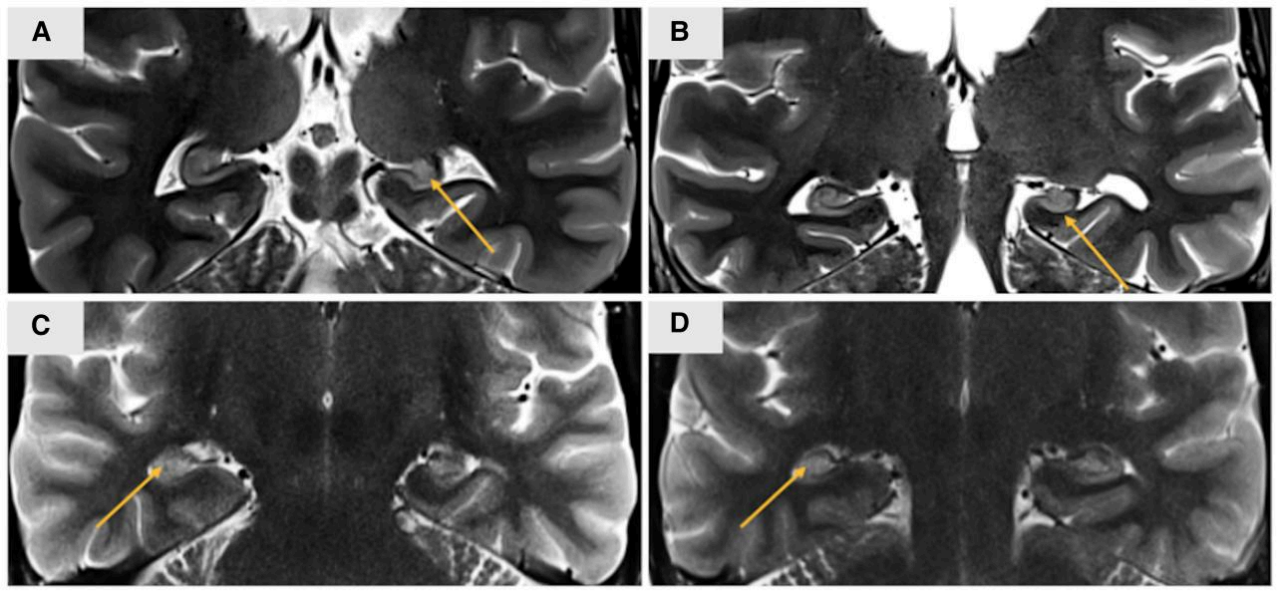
MRI coronal T2-weighted images for two monozygotic twin pairs with neurofibromatosis type 1 who were concordant for hippocampal sclerosis. (A and B) Pair 1. (C and D) Pair 2. In each case, both hippocampi were abnormal; however, there was a lateralized emphasis. The hippocampus ipsilateral to the TLE in each twin met the diagnostic criteria for hippocampal sclerosis (HS), while the contralateral hippocampus displayed a high T2 signal. Pair 1: (A) left HS (arrow). This twin also had an increased T2 signal in the posterior body of the right hippocampus (not shown). (B) Left HS (arrow) and high T2 signal in the right hippocampus. Pair 2: (C) right HS (arrow) and high T2 signal in the left hippocampus. (D) Right HS (arrow) and increased T2 signal in the left hippocampus. TLE = temporal lobe epilepsy.
